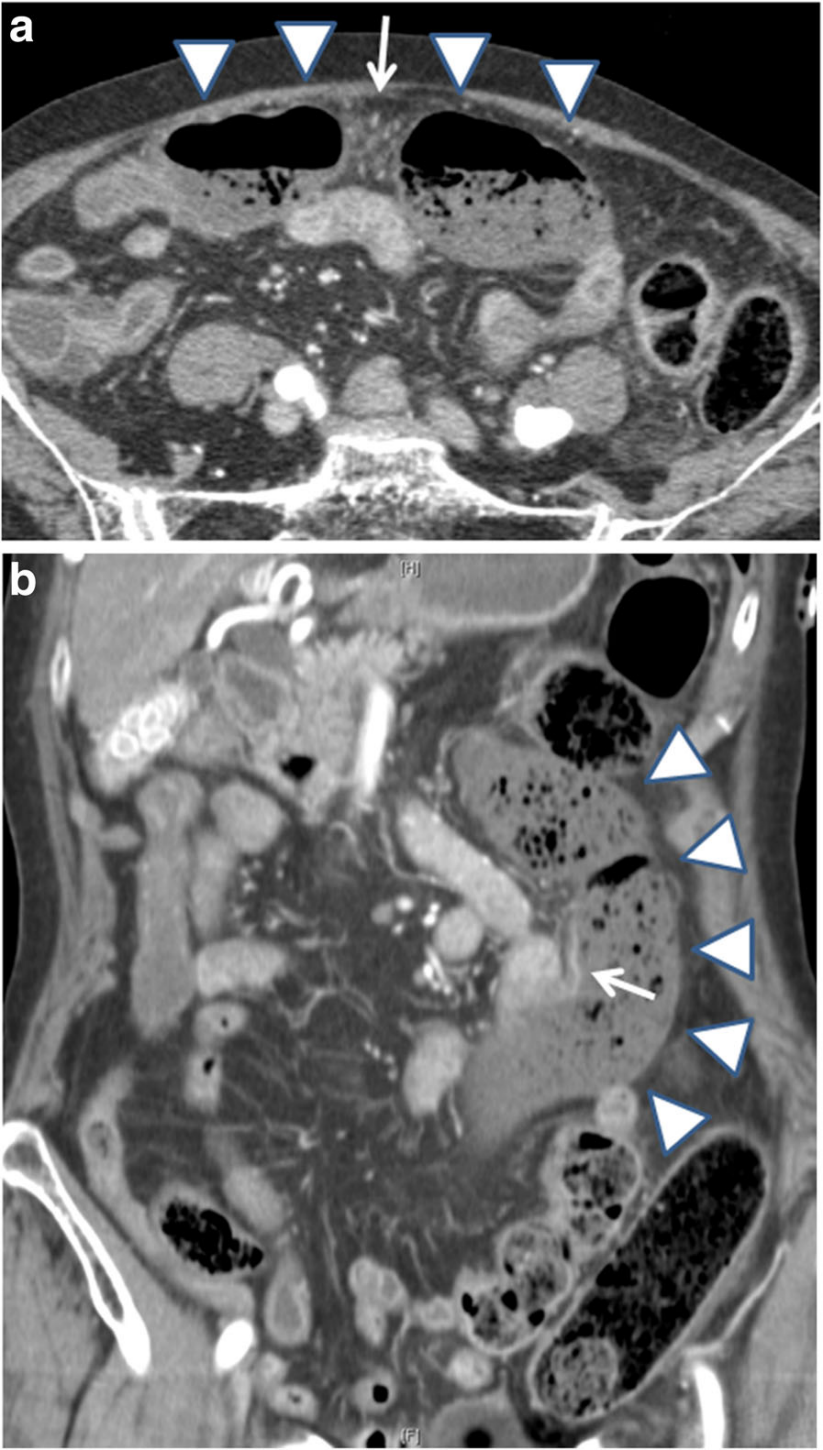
Contrast-enhanced computed tomography scan. a The dilated and thinned transverse colon was detected in the axial view. The enhancement of peripheral artery was not attenuated (*arrow*). b Remaining partial enhancement of the transverse colon (*arrow*). The enhancement of the other part of the transverse colon was attenuated
